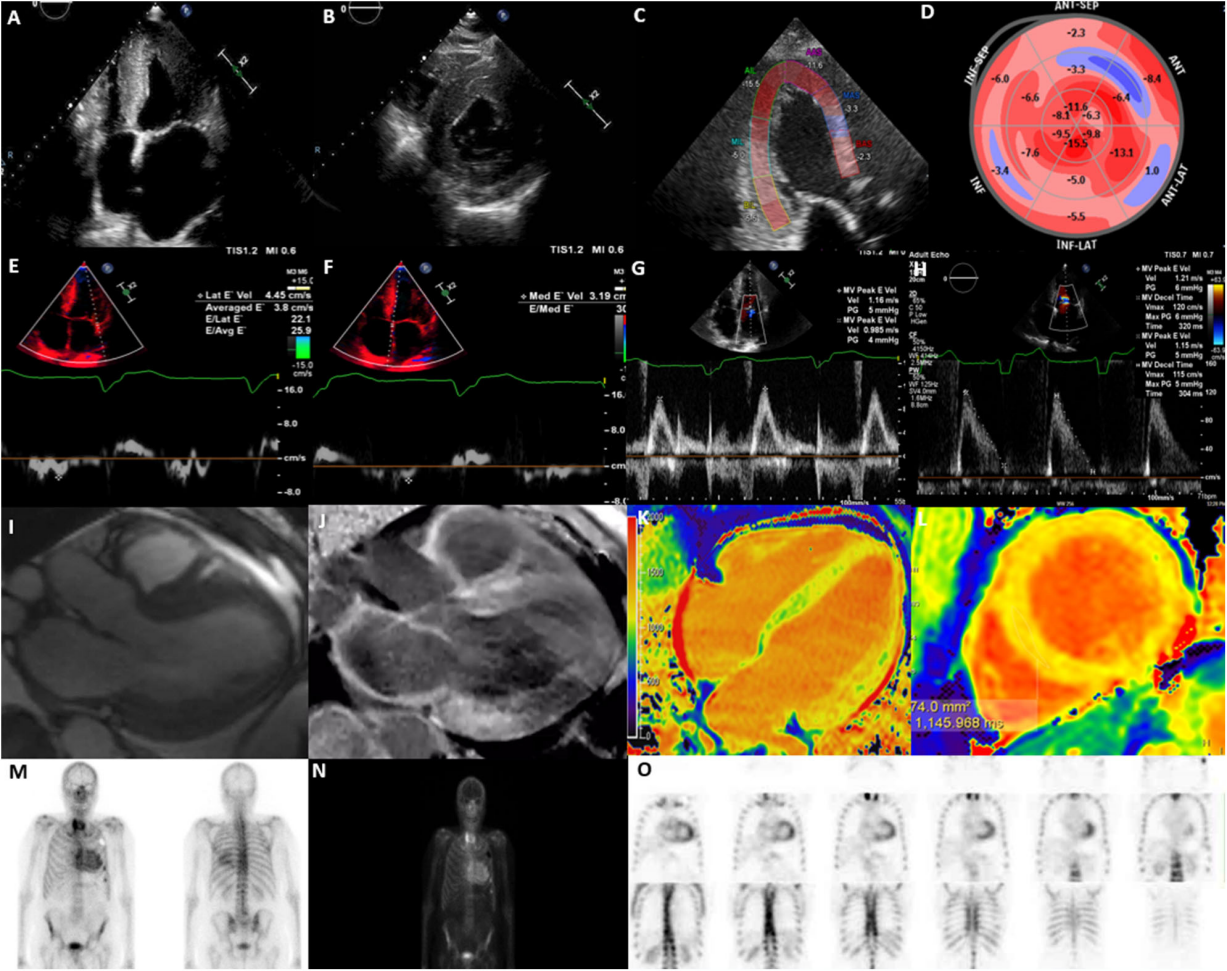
Multi-modality imaging in cardiac amyloidosis. (A) Apical 4-chamber view showing increased left ventricular wall thickness (16 mm), dilated left atrium and myocardial speckling. (B) Parasternal short-axis view showing increased left ventricular wall thickness. (C) Global longitudinal strain on apical 3-chamber view showing presence of apical-sparing. (D) Bull's eye longitudinal strain showing evidence of apical-sparing. (E) Lateral E' Doppler velocity of 4.45 cm/s (N>10 cm/s). (F) Medial E' Doppler velocity of 3.19 cm/s (N>7 cm/s). (G) Mitral inflow with an MV peak E velocity of 1.16 m/s (N: 0.6–0.8 m/s). (H) Mitral inflow showing a reduction in deceleration time. (I) Cine CMR axial 3-chamber view showing concentric increase in left ventricular wall thickness and atrial dilatation. (J) Cine CMR axis 3-chamber showing evidence of widespread late gadolinium enhancement, particularly affecting basal inferior, basal anterior left ventricle and left atrium. (K) T1 mapping (Modified inversion recovery Look-Locker [MOLLI 3(3)3(3)5] 4-chamber view showing increase in left ventricular native T1 time (region of interest; mid-septum) consistent with amyloidosis; (L) T1 mapping MOLLI [3(3)3(3)5)] short-axis view, showing increase in left ventricular mean native T1 time (region of interest; mid septum) consistent with amyloidosis [T1 = 1,145 ms (*N* = 930–1,000 ms)]. (M) Bone Tracer Cardiac Scintigraphy using Tc99-DPD showing increased myocardial activity in the ventricles consistent with TTR amyloidosis. (N) Whole body 3 h sweep showing increased myocardial activity in the ventricles consistent with TTR amyloidosis; (O) Bone Tracer Cardiac Scintigraphy using Tc99-DPD showing increased myocardial activity in the ventricles consistent with TTR amyloidosis. Perugini score is 3 correlating with strong cardiac uptake with mild or absent bone uptake.
